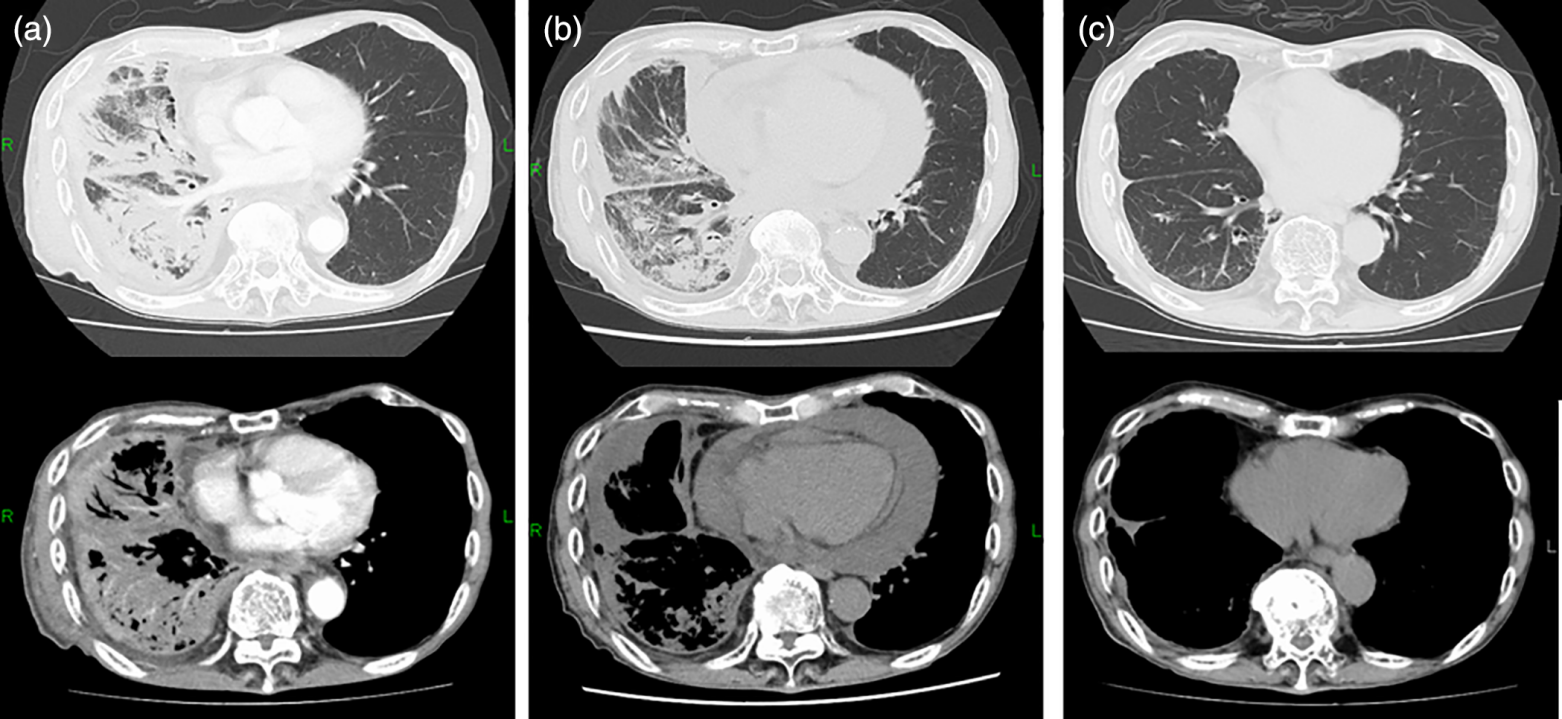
Chest computed tomography (CT). (A) Prior to treatment with pembrolizumab. CT showed carcinomatous lymphangitis and pleural effusion. The primary site was not clear under cover to lymphangitis. (B) After six cycles. A massive pericardial effusion was observed. The other malignant lesions improved. (C) After 11 cycles. The patient showed a continuous good response.
